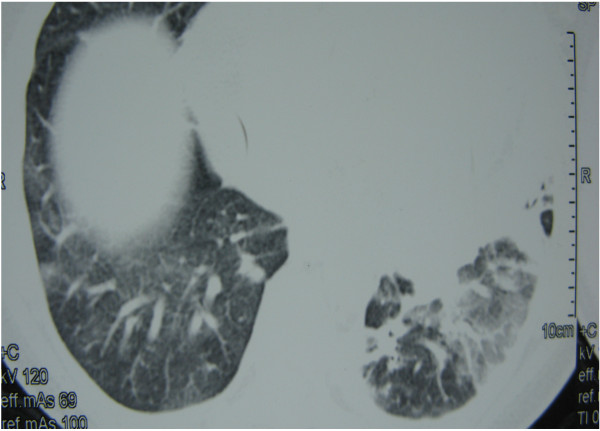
Chest CT showing bilateral subpleural nodules and left basal ground glass pattern.
